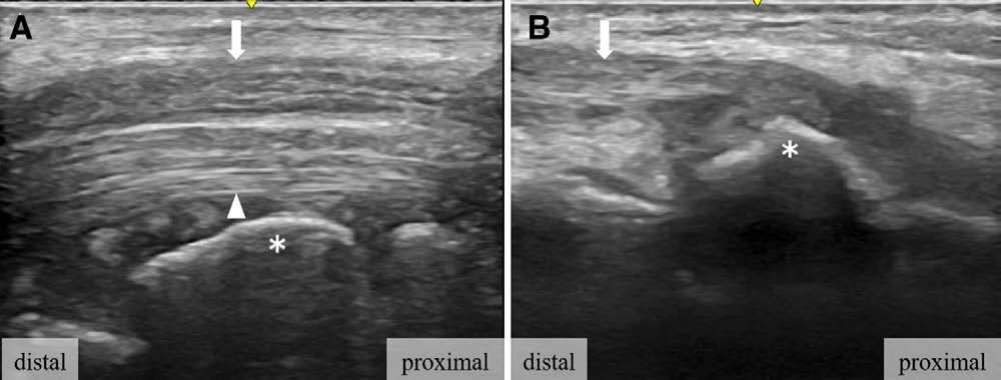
Ultrasound findings. (A) Longitudinal view to the volar aspect of wrist showing volar segment of lunate bone (asterisk) that does not interfere with the median nerve (arrow) or flexor tendon (arrowhead). (B) Longitudinal view to the dorsal aspect of wrist showing distal stump of ruptured extensor tendon (arrow) and dorsal segment of lunate bone (asterisk).
